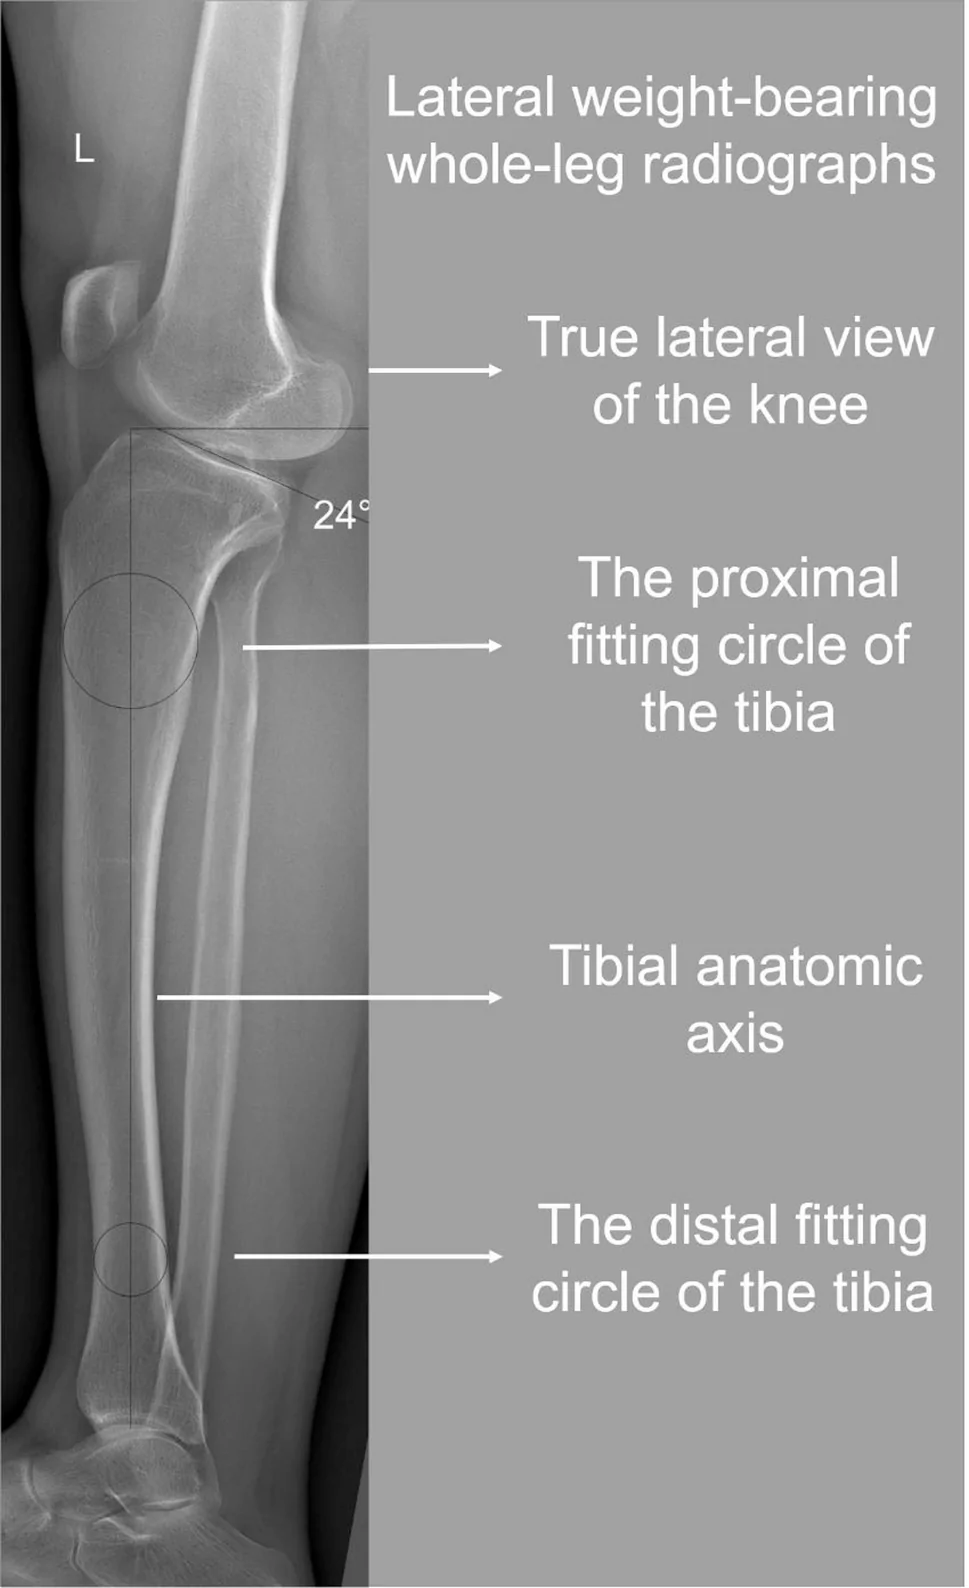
Measurement of the PTS [18]. On true lateral radiographs of the lower limb, fitting circles are made at the proximal and distal cortices of the tibia to determine the TAA. The angle between the tangent to the medial tibial plateau and the line perpendicular to the TAA is defined as the PTS
